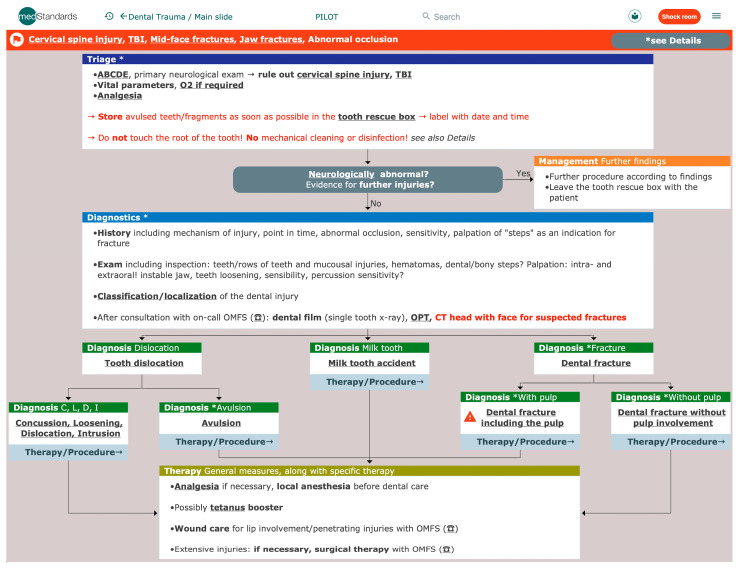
Screenshot ”medStandards” [16]—Dental Trauma. ABCDE: mnemonic for primary survey; TBI: traumatic brain injury; O_2_: oxygen; OPT: orthopantomogram; CT: computed tomography; OMFS: oral and maxillofacial surgeon.
